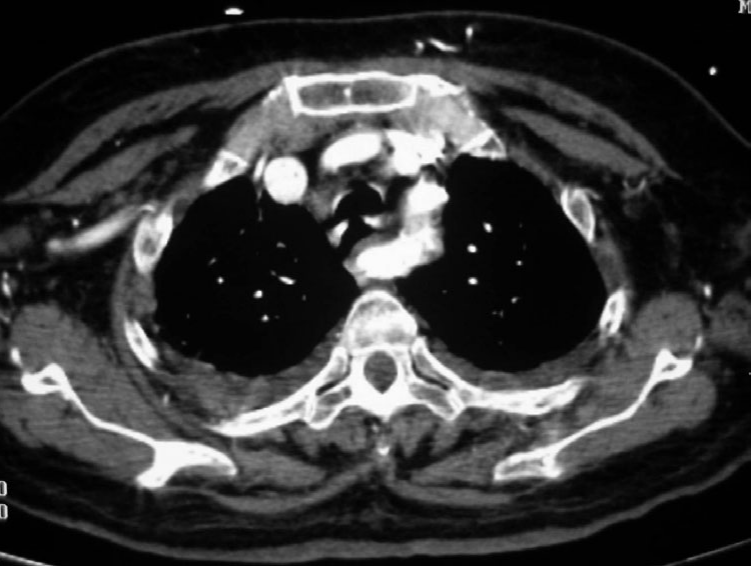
Anomalous origin on the right subclavian artery. Serial images from a helical CT scan of the superior mediastinum, cranial to caudal. Notice the large enhancing vascular structure posterior to the esophagus (*). On the more caudal images, a direct origin of this vessel from the aortic arch, distal to the origin of the left subclavian artery, is noted.
